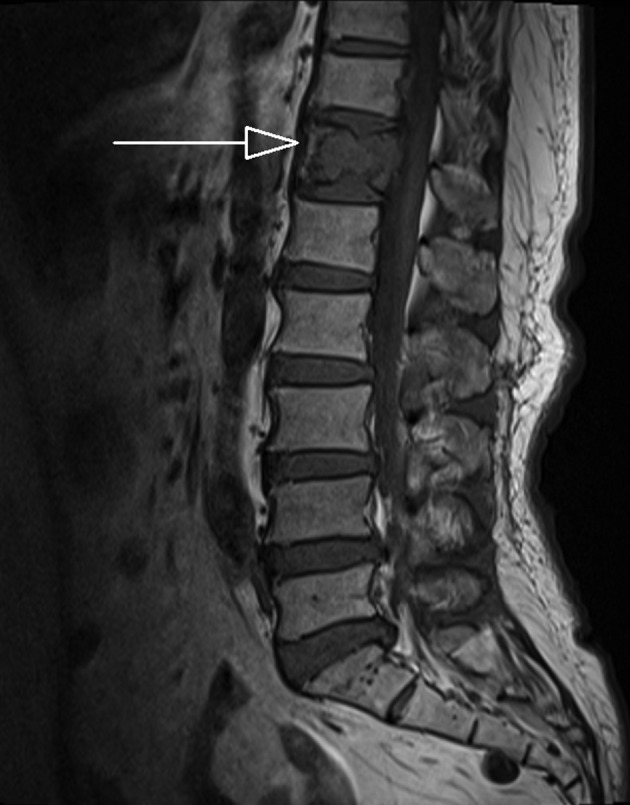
Magnetic resonance imaging showing the 12th thoracic vertebra with bony destruction.
